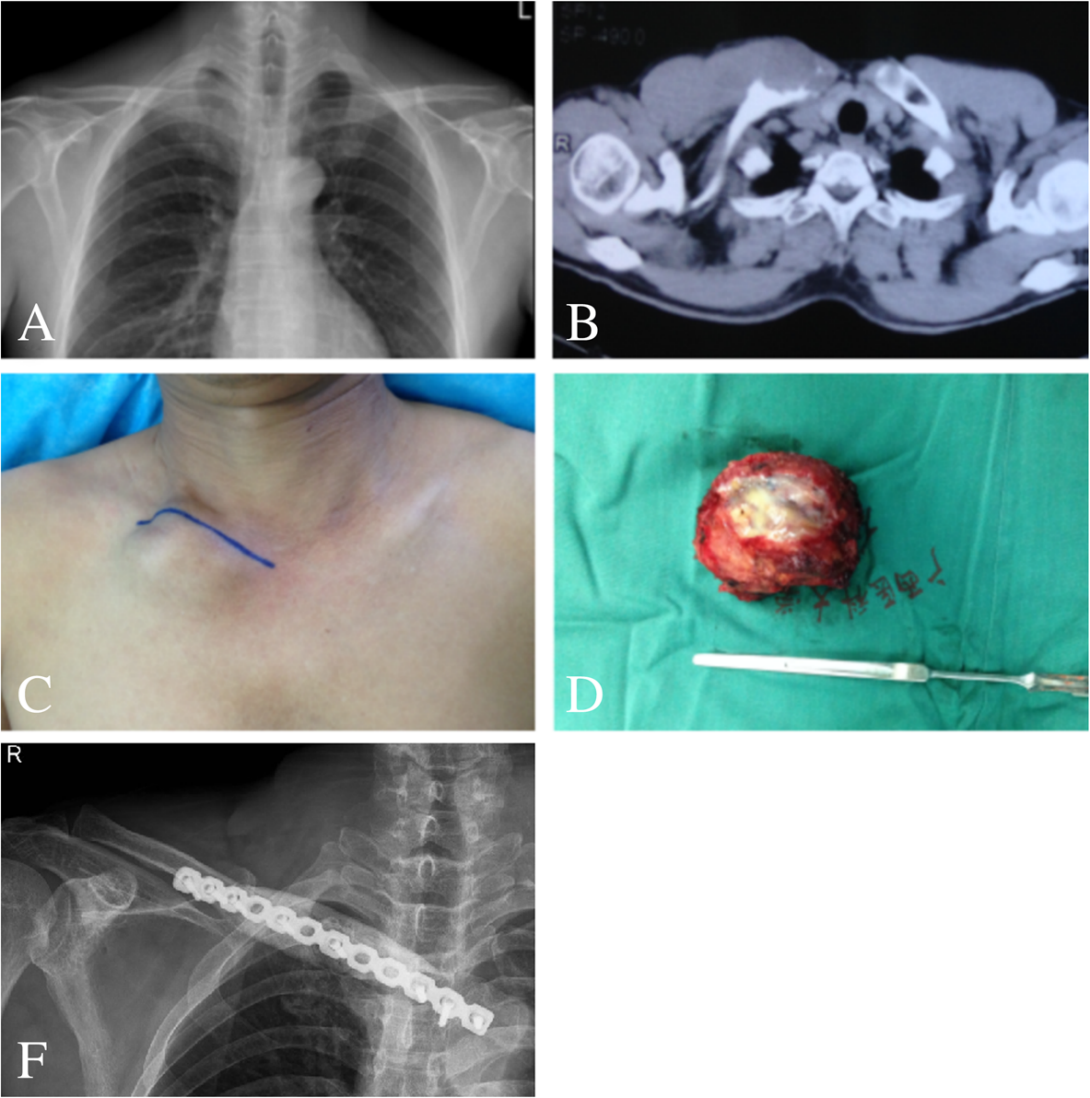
A 46-year-old man with right, medial, and clavicular synovial sarcoma. a, b Preoperative plain X-ray and CT show an obvious tumor destruction on the medial right clavicle. c, d General view of the tumor during surgery. f Postoperative X-ray shows the plate-cement complex is well fixed
